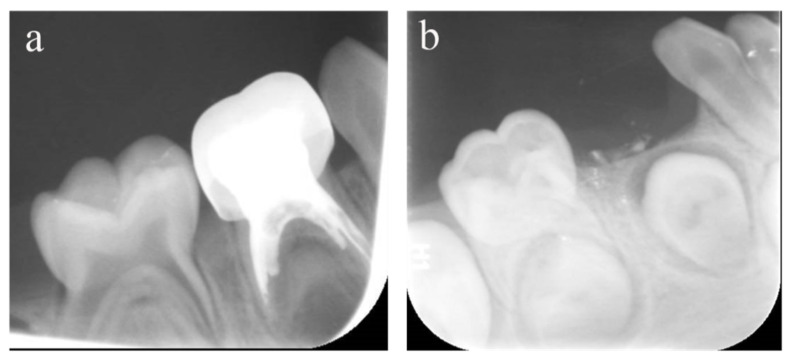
#84 was treated with pulpectomy and filled with ZOE under DGA. The follow-up period was 34 months (31-month-old male child) (a) Two weeks after the operation, the radiographic showed that the filling in the distal roots was underfilled. (b) Thirty-four months after treatment, #84 was lost early, but a portion of the ZOE particles remained. The pulpectomy in #84 failed.
